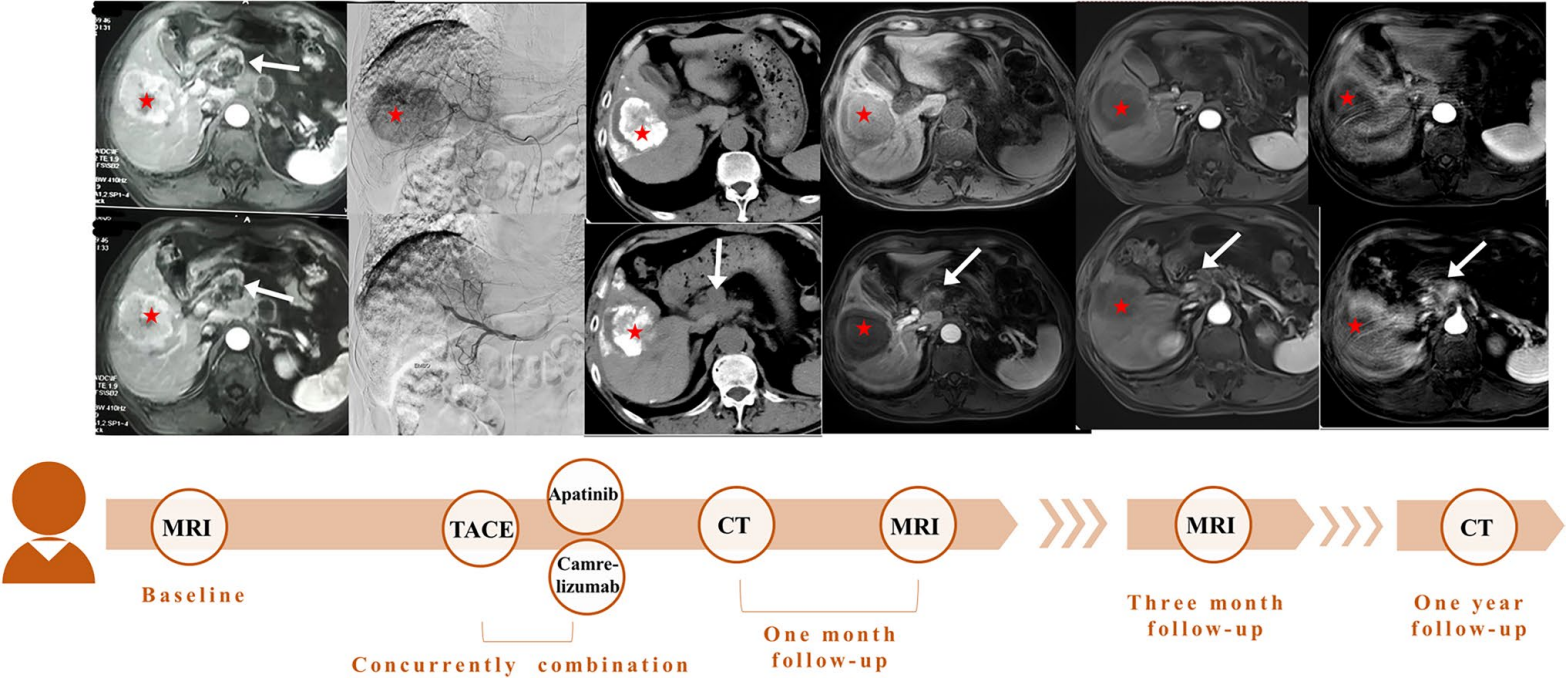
A 72-year-old man had a history of chronic hepatitis B for more than twenty years. The baseline MRI showed that there is a typical HCC lesion (red asterisk) in the right liver lobe with intrahepatic metastasis. The enlargement of the porta hepatic lymph node (white arrow) was considered an extrahepatic spread malignancy. Then, the patient received TACE combined with camrelizumab (200 mg, ivgtt, Q3w) and apatinib (250 mg, po, QD). The first, three-month, 1-year follow-up imaging after combination therapy showed a partial response. MRI, magnetic resonance imaging; CT, computed tomography; HCC, hepatocellular carcinoma; TACE, transarterial chemoembolization
